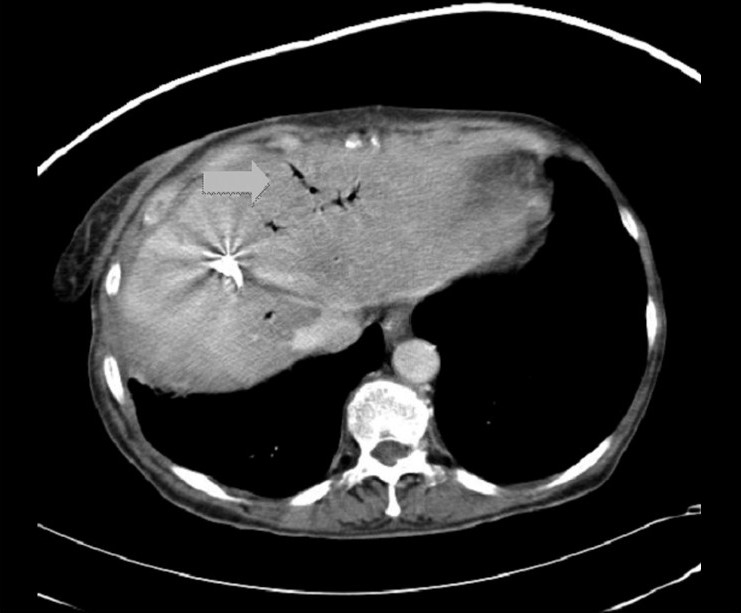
CT of the abdomen prior to drain removal demonstrating both healing of the liver laceration and resolution of the intra-hepatic infection; however, pneumobilia persists
